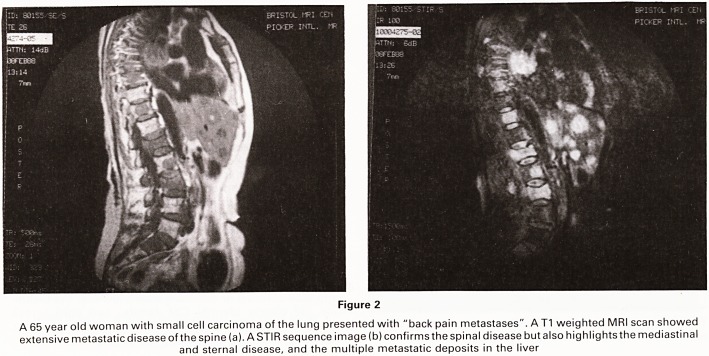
# The Detection of Metastatic Disease of the Spine in Oncology Patients Using MRI
